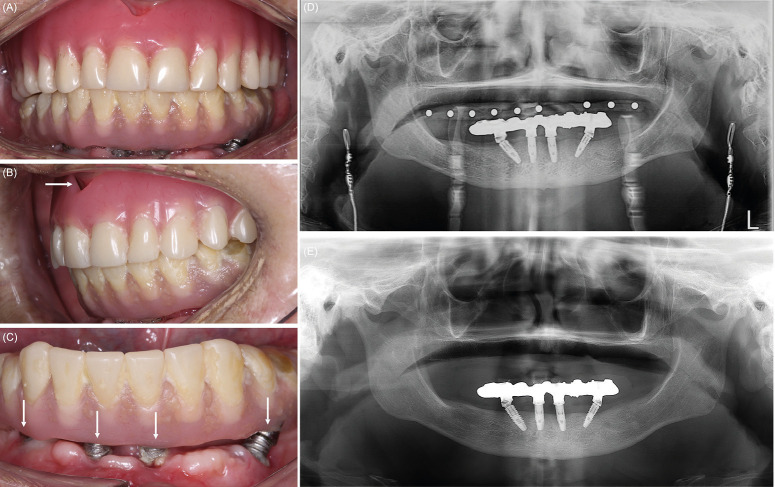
(A) frontal view show a upper complete removable denture, and a lower implant-supported fixed complete hybrid denture; (B) lateral show an ill-fitting denture with a notorious gap (horizontal white arrow) between the residual ridge and the denture base; (C) close-up view of the implant-supported fixed denture showing exposure of the implants platforms and threads (vertical white arrows), dental plaque around implants, and a notorious gap between lower prosthesis and residual mucosa; (D) immediate after-treatment and (E) current panoramic radiographs that evidence a generalized horizontal bone loss and signs of periimplantitis.
